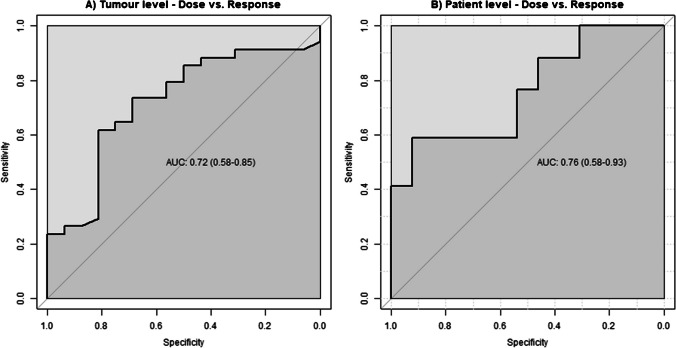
A Receiver operating characteristic curves (ROCs) showing discriminative value of tumour absorbed dose for response based on SPECT; AUC is 0.72 (95% CI: 0.58–0.85). B Ability of mean tumour absorbed dose per patient to discriminate between patients with complete or partial response and progressive or stable disease-based AUC is 0.76 (95% CI: 0.58–0.93). ROCs are not based on clustered data analysis, but area-under-the-curves (AUCs) are
